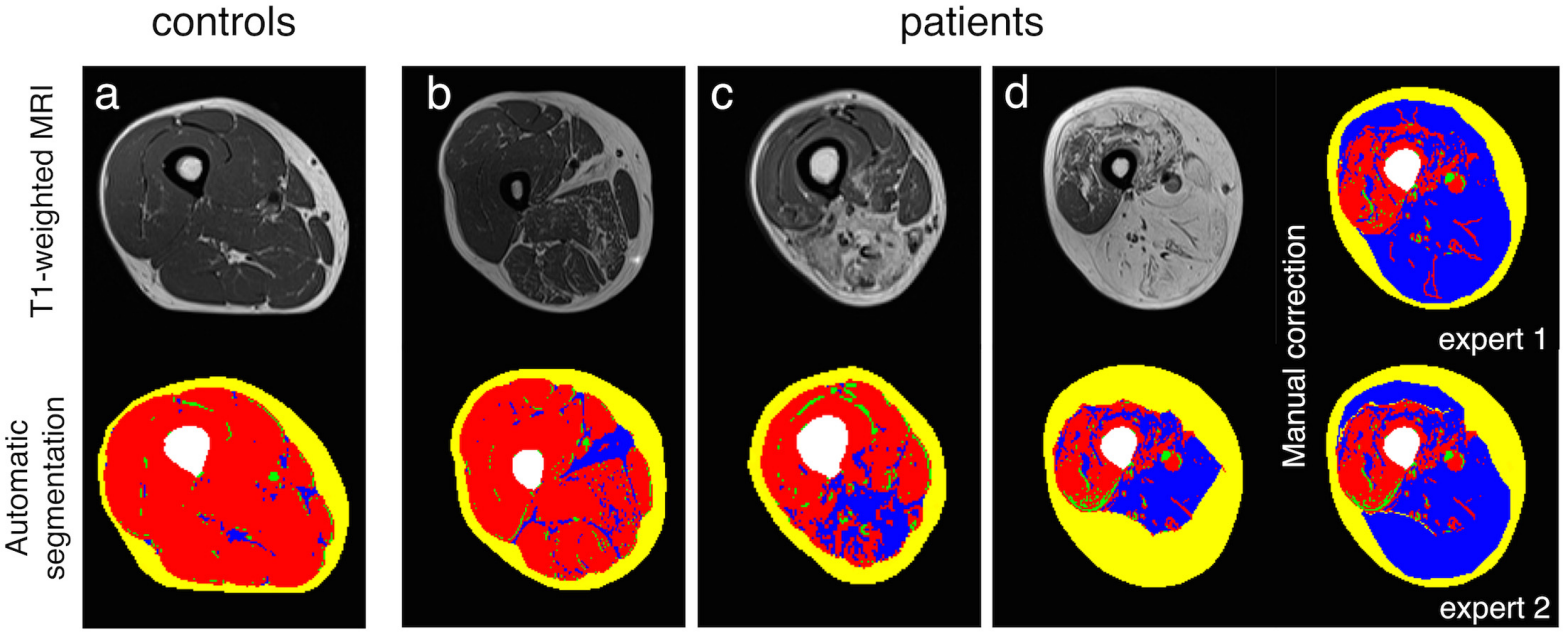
Examples of MR images. Examples of a successful automated segmentation in a control subject (a) and two patients (b and c). Examples of MR images with manual correction of the segmentation process (d).
